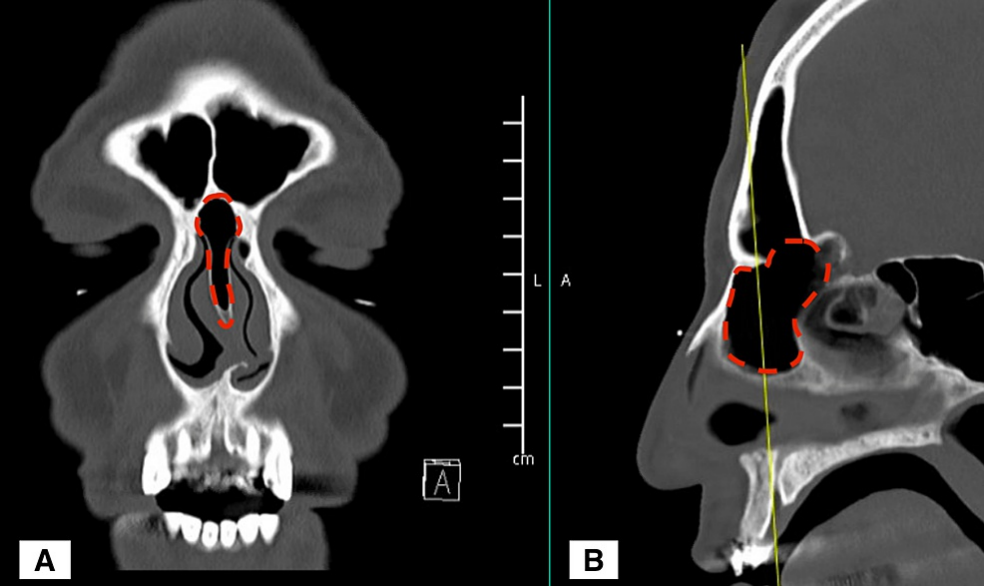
CT scan of the paranasal sinus; A: coronal cut; B: sagittal cut The area marked in red represents the Septo-Frontal Cell.
